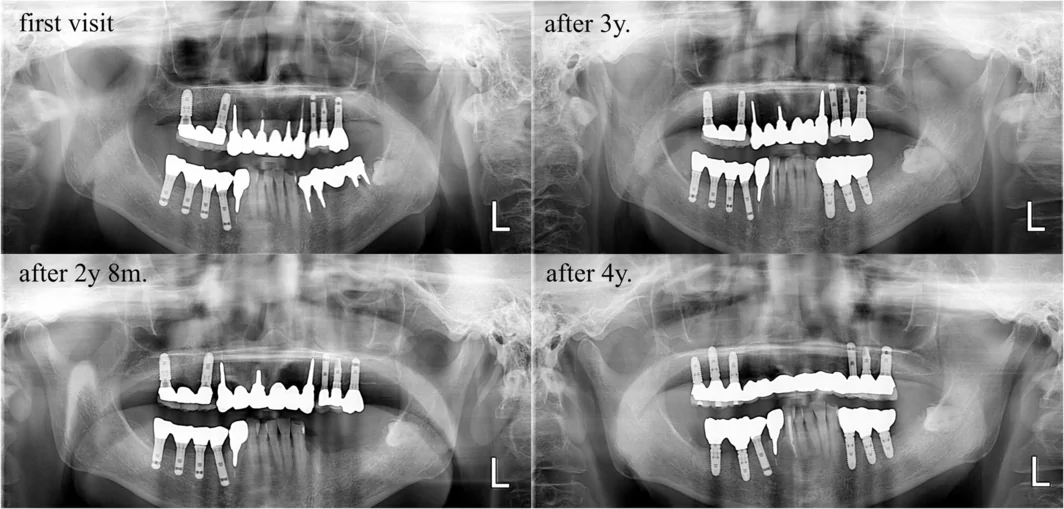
Serial panoramic radiographs from baseline, surgical intervention, and prosthetic completion.
